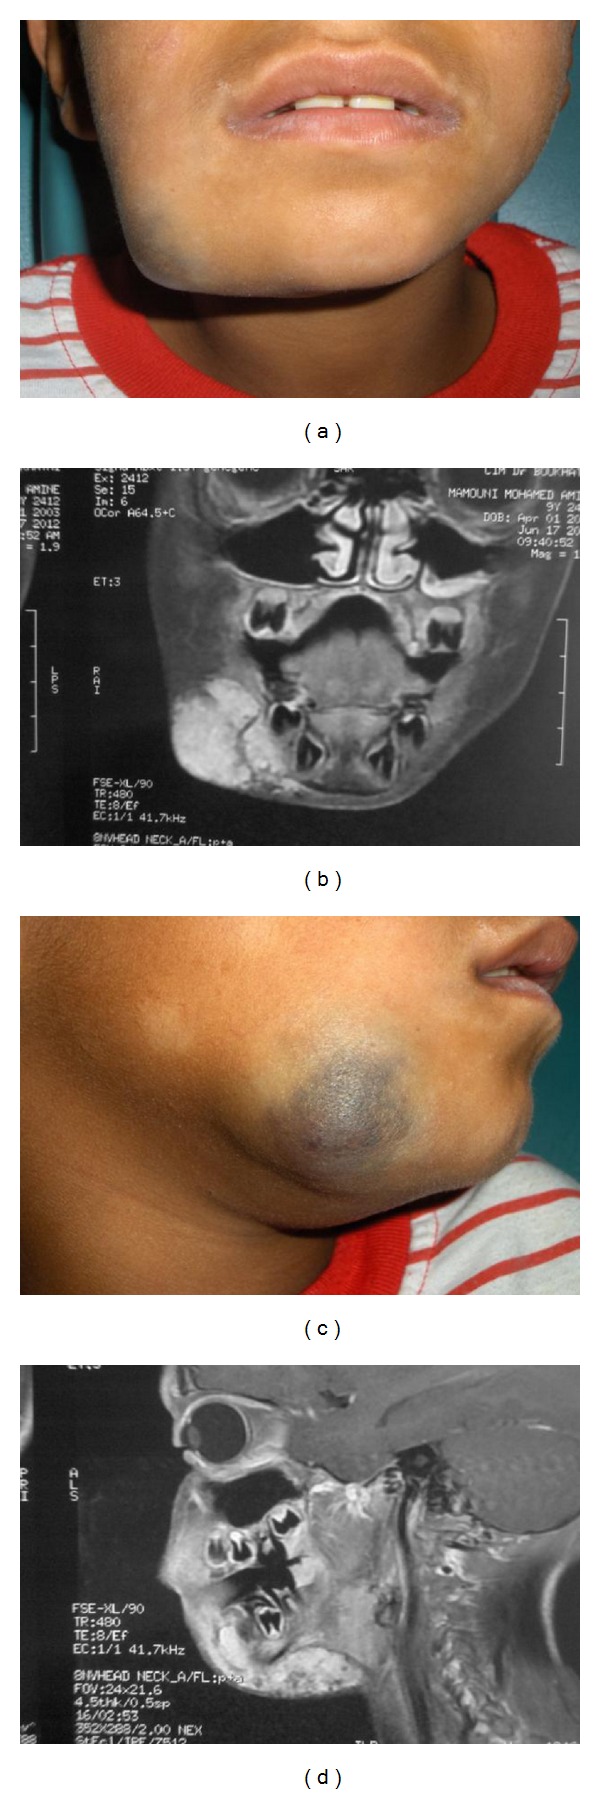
A magnetic resonance imaging (MRI) of superficial and deep venous malformation. The technique was performed in three planes with T2 fat-saturation (T2-FS), axial T1, 2D time-of-flight (2D TOF), magnetic resonance angiography (MRA) of supra-arterial trunks, and three planes T1-FS for the cervical region.
